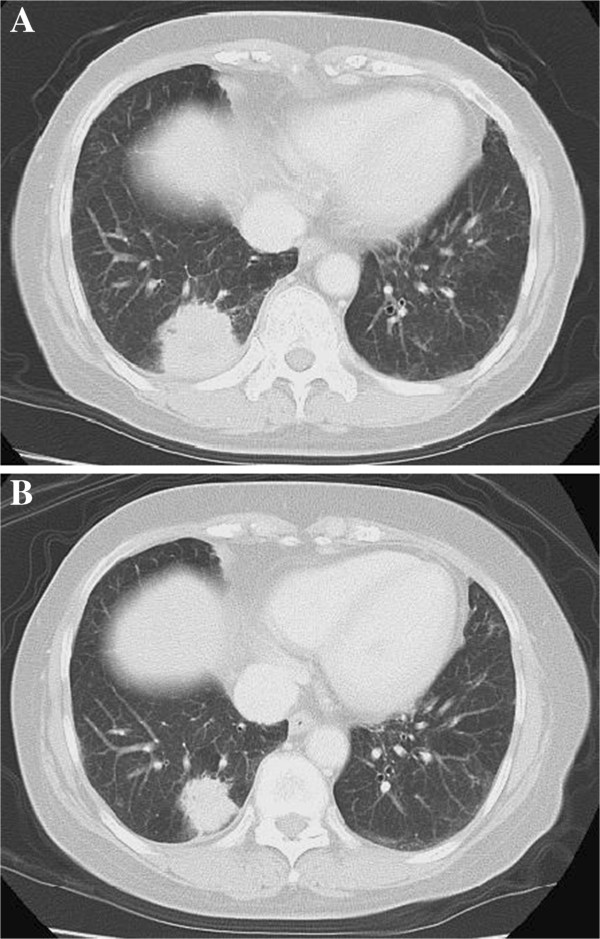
Gastric lesion observed by gastroscopy before and after treatment with carboplatin plus pemetrexed. ( A) Computed tomography scans before treatment showing the tumor at a maximal diameter of 36mm with an irregular border in segment 10 of the right lung and ( B) shrinking tumor judged as a partial response after four cycles of carboplatin plus pemetrexed.
